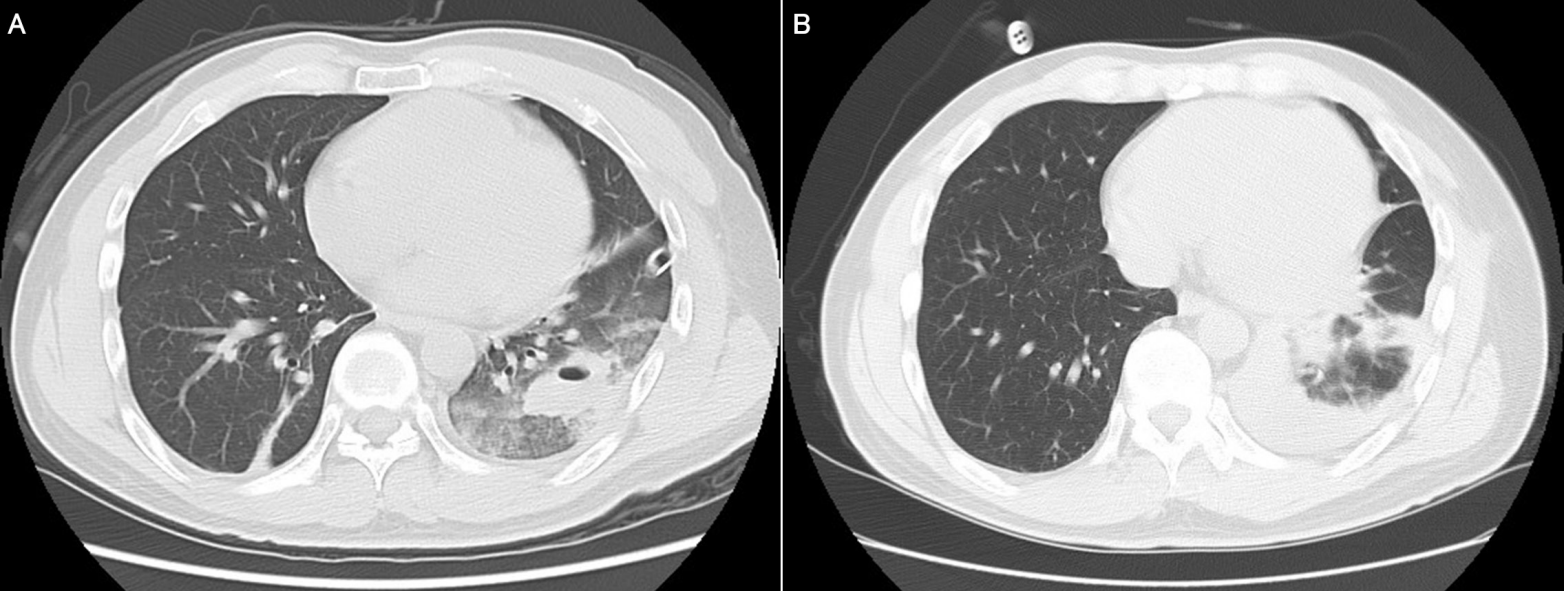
(A) Chest CT at the time of the first accident (10 years before presentation) showing pulmonary contusion and traumatic pneumatocele in the left lower lobe. No significant traumatic changes were observed in the other lobes. (B) Chest CT at the time of the second accident (6 months after the first accident) showing left hemothorax and subpleural infiltrates in the left lower lobe, while the previous pulmonary contusion and traumatic pneumatocele had resolved, with no significant abnormalities in the other lobes.
